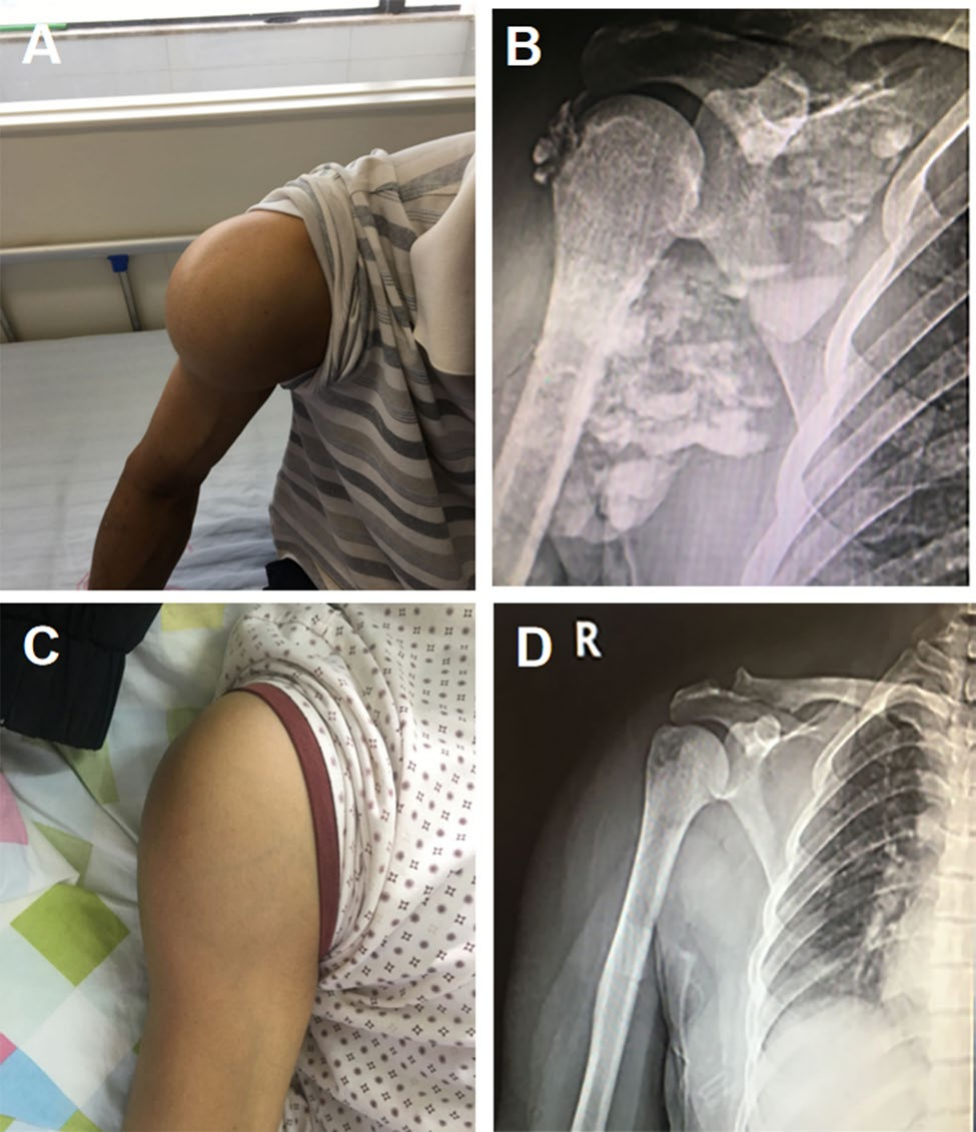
Changes in the Right Shoulder Mass Over Time. A, B) Gross appearance and X-ray imaging of the right shoulder mass in 2018. C, D) Gross examination and X-ray imaging of the right shoulder five months post-PTX
